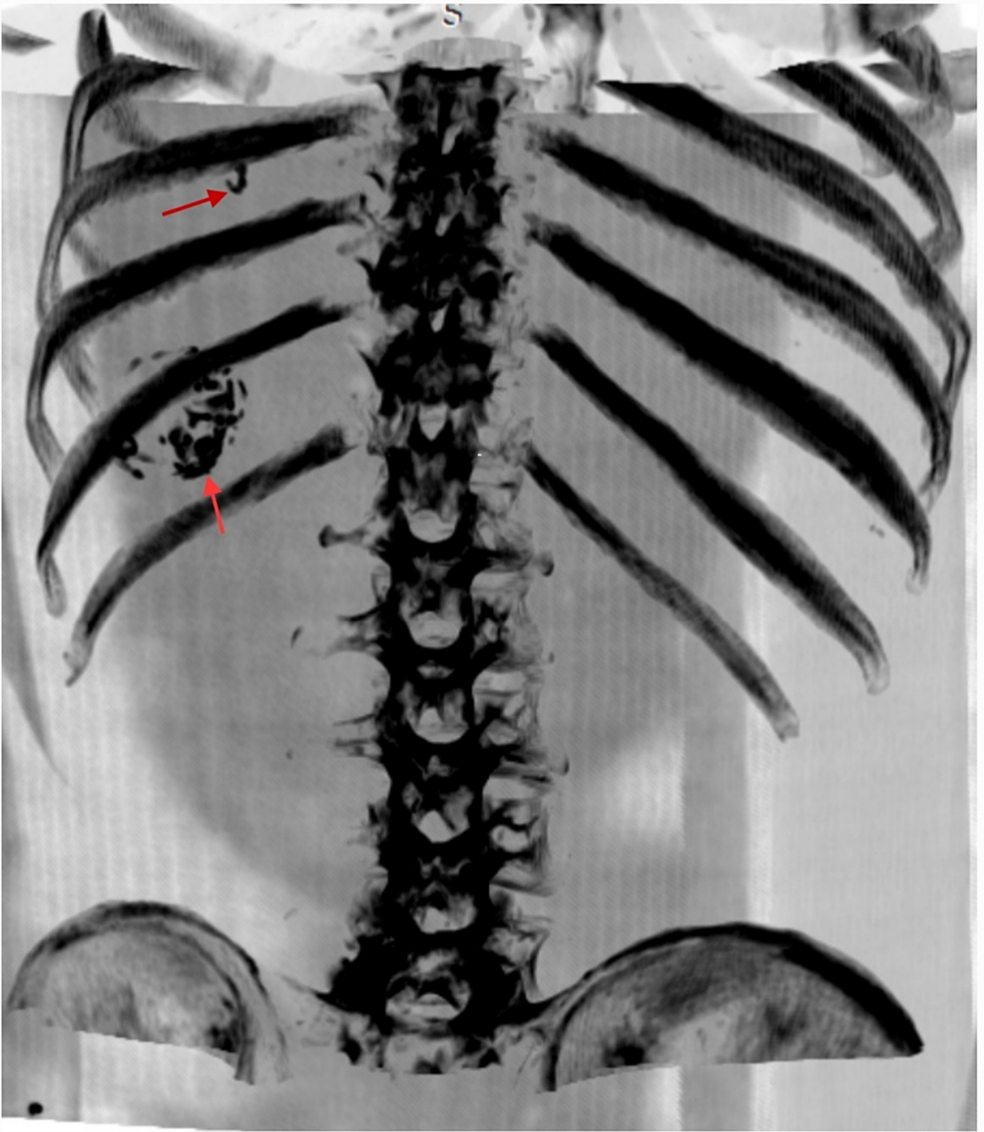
Maximum intensity projection (MIP) image showing inactive cyst. MIP image showing inactive cyst with circumferential calcification at the level of 11th rib. Another incomplete ring of calcification is seen at the level of ninth rib (which is a part of primary cyst and indicates active hydatid cyst). An important point, which is to be noted here, is that due to transdiaphragmatic spread of the hepatic hydatid into the adjacent lung it can mimic pulmonary hydatid on chest x-ray but suspicion can raise when the pulmonary hydatid is calcified, which is extremely rare. So, for any case of calcified pulmonary hydatid cyst further evaluation is to be warranted to look for transdiaphragmatic spread of hepatic hydatid.
